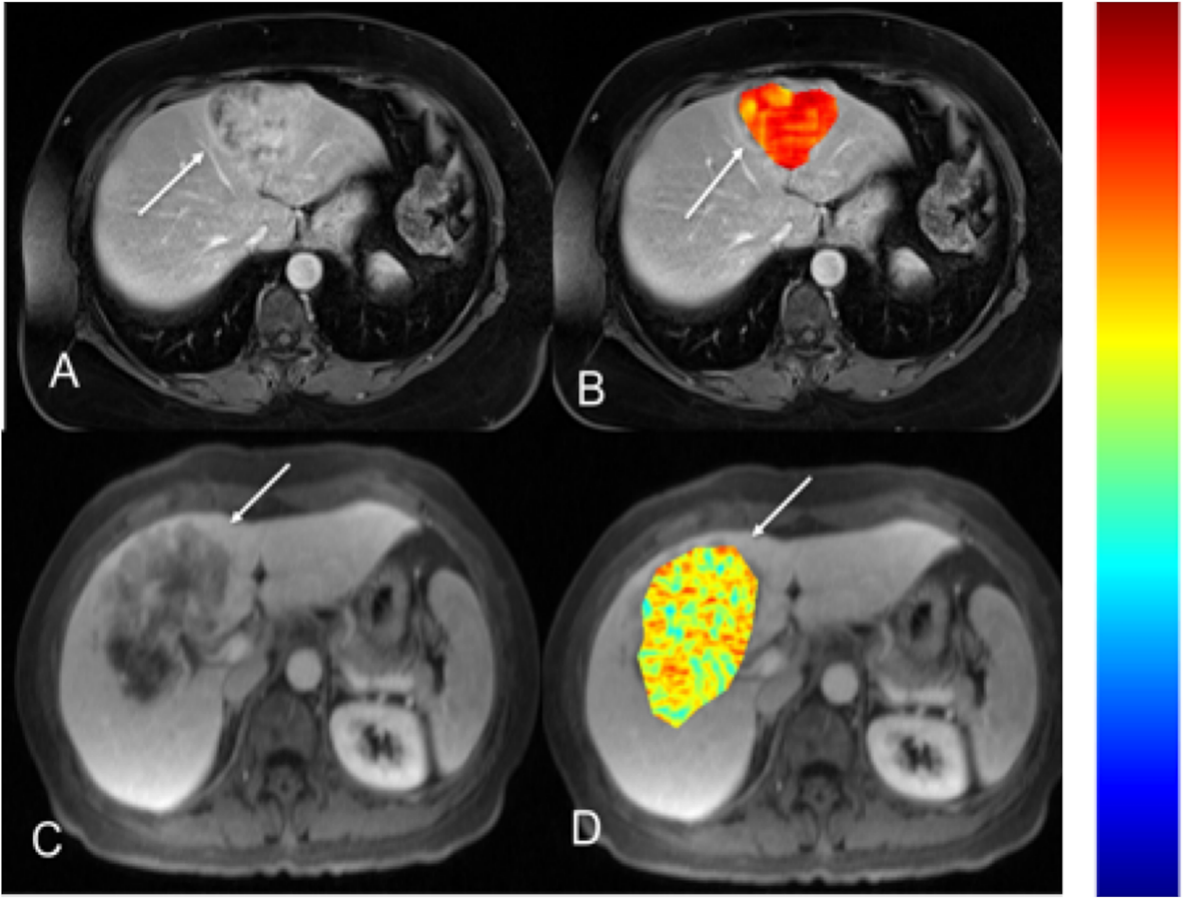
MRI texture feature of difference entropy found to be a significant predictor of TTR on univariable analysis. Top row (a, b): 54-year-old female with moderately differentiated ICC in segment 4 (AJCC stage 1). T1-weighted post-contrast MR image obtained during portal venous phase (a, arrow) with an overlying difference entropy texture map (b, arrow). This patient’s ICC difference entropy parameter was calculated to be 2.16 (scale 0–3, blue-red), with corresponding TTR of 825 days. Bottom row (c, d): 56-year-old female with poorly differentiated ICC (AJCC stage 3) in segment 4/8 on T1-weighted post-contrast MR image obtained during portal venous phase (c, arrow) and with an overlying difference entropy texture map (d, arrow). This patient’s ICC difference entropy parameter was calculated to be 1.22 (scale 0–3, blue-red) with corresponding TTR of 456 days. Color scale bar is shown on the right
